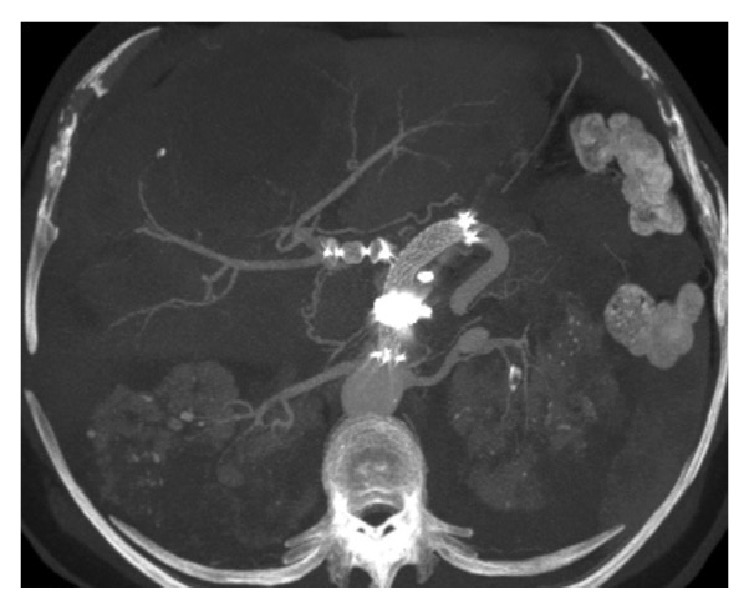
CT scan performed 30 days after the procedure confirming procedural success. MIP reconstruction: complete exclusion of the aneurysm with patency of the Fluency stent in the splenic artery and occlusion of the common hepatic artery with Amplatzer Vascular Plug and Onyx to fill the aneurysm sac. A 1.3 cm of the left renal artery is recognizable.
